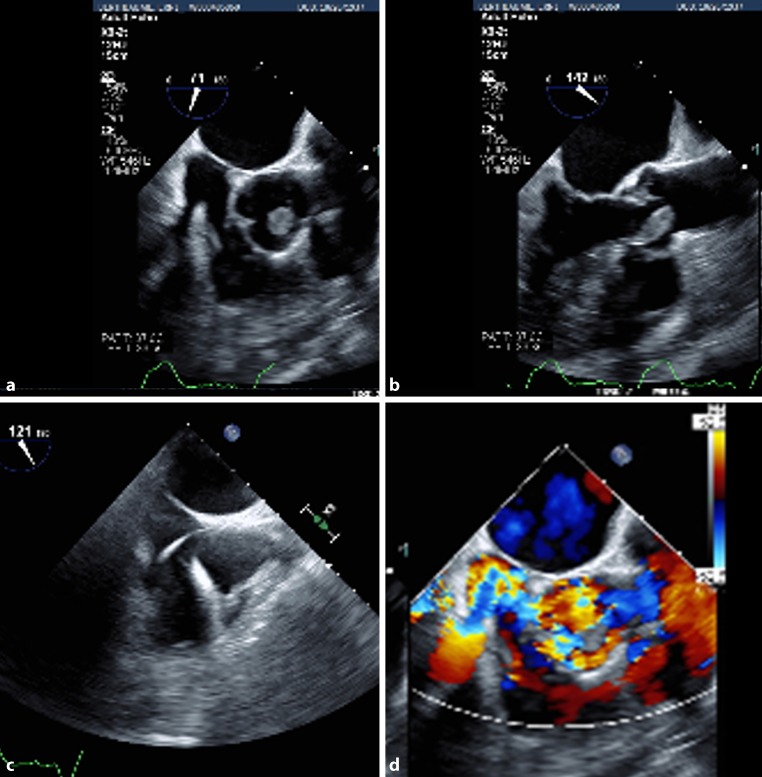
a Short axis view and b long axis view at the level of mid oesophagus showing 1.5 × 1.4 cm echo-dense structure on the right coronary cusps of the aortic valve. Upper oesophageal view c showing right atrial pacer lead with thin filamentous 1‑cm long freely mobile echo-dense structure and d Doppler showing severe aortic regurgitation
